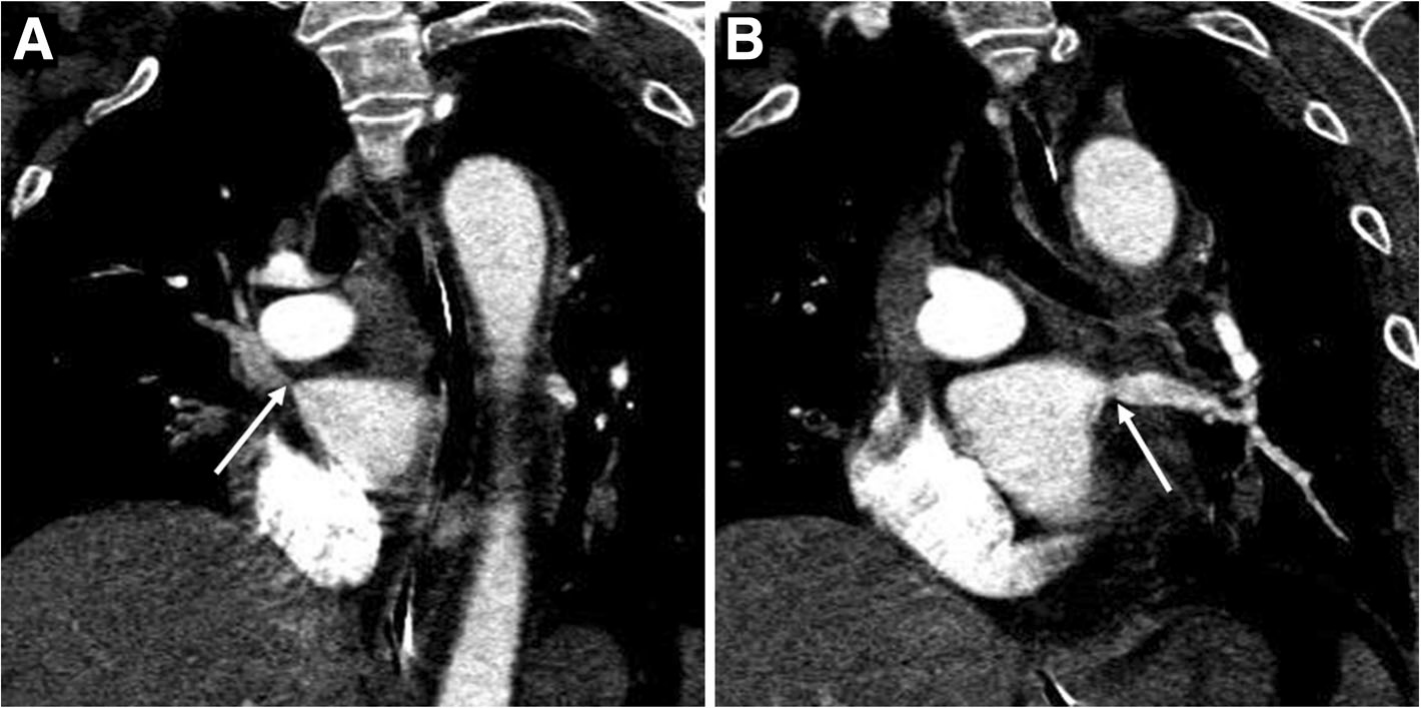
a Oblique coronal maximum intensity projection computed tomography (CT) showing stenosis (approximately 95%, *arrow*) of the right superior pulmonary vein (PV). b Oblique coronal maximum intensity projection CT showing stenosis (approximately 90%, *arrow*) of the left inferior PV
